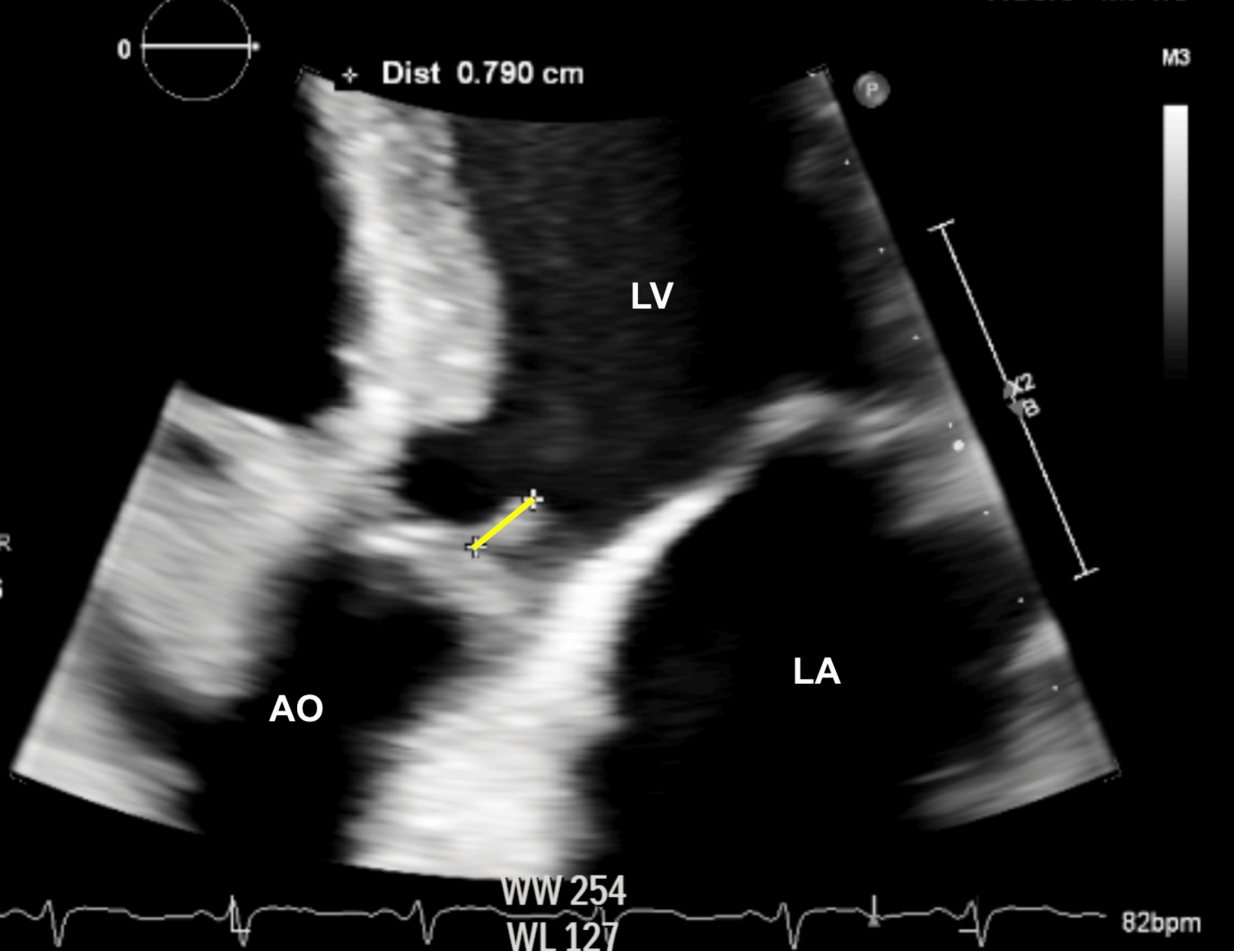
Transthoracic echocardiogram (TTE) displaying 0.79 cm vegetation on the aortic valve (yellow line). LA: left atrium; LV: left ventricle; AO: aorta.
